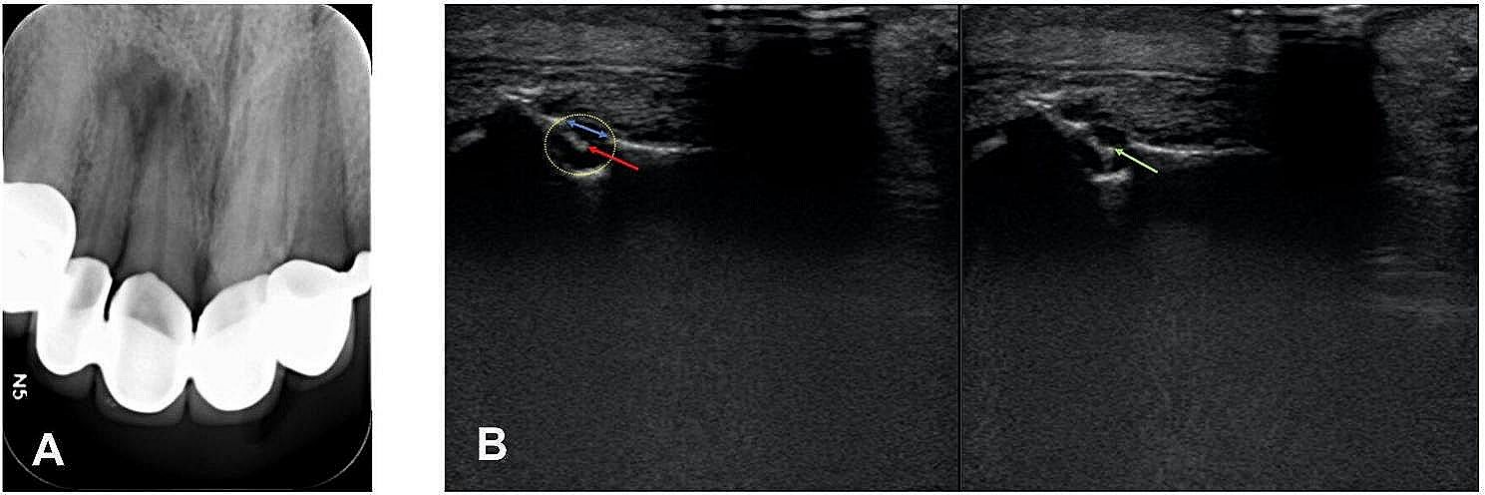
(A) A periapical image of a right central and lateral tooth with apical radiolucency. (B) Ultrasound image of the same patient, captured in real-time with a canal file inserted into the root canal. In the image, the green line represents the brightly visible tip of the canal file instrument, while the red line marks the root apex, appearing white but darker than the instrument tip. The blue line indicates the region of resorption on the cortical bone corresponding to the teeth
